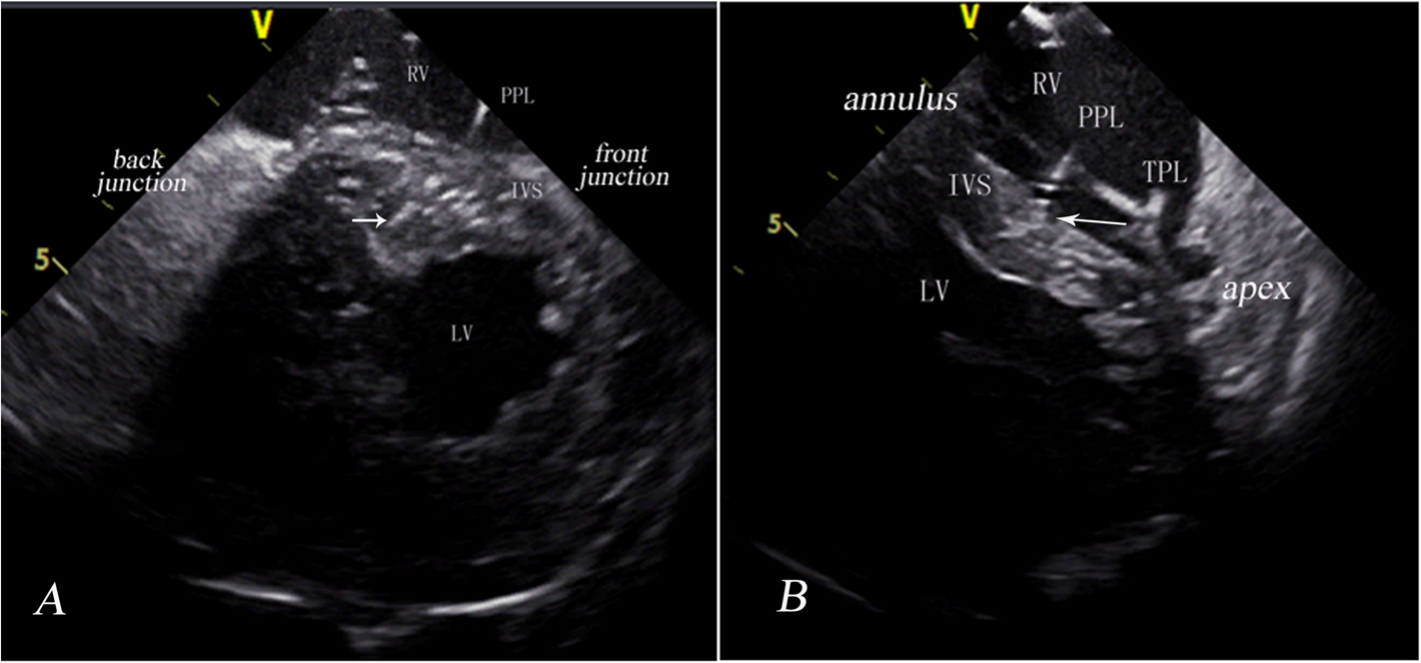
Intracardial echocardiography views obtained with the catheter in the RV. a: Short axis view of the septum around the mitral valve plane. The distance between the implantation site and the front junction point and the distance between the implantation site and the back junction point should be measured. b: Longitudinal view of the septum. The distance from the implantation site to the apex and the distance from the implantation site to the tricuspid annulus should be measured. RV, right ventricle; LV, left ventricle; V, transducer location; IVS, interventricular septum; 5, distance to the transducer; PPL, permanent pacing lead; TPL, temporary pacing lead; short arrow, lead tip; long arrow, implantation site
